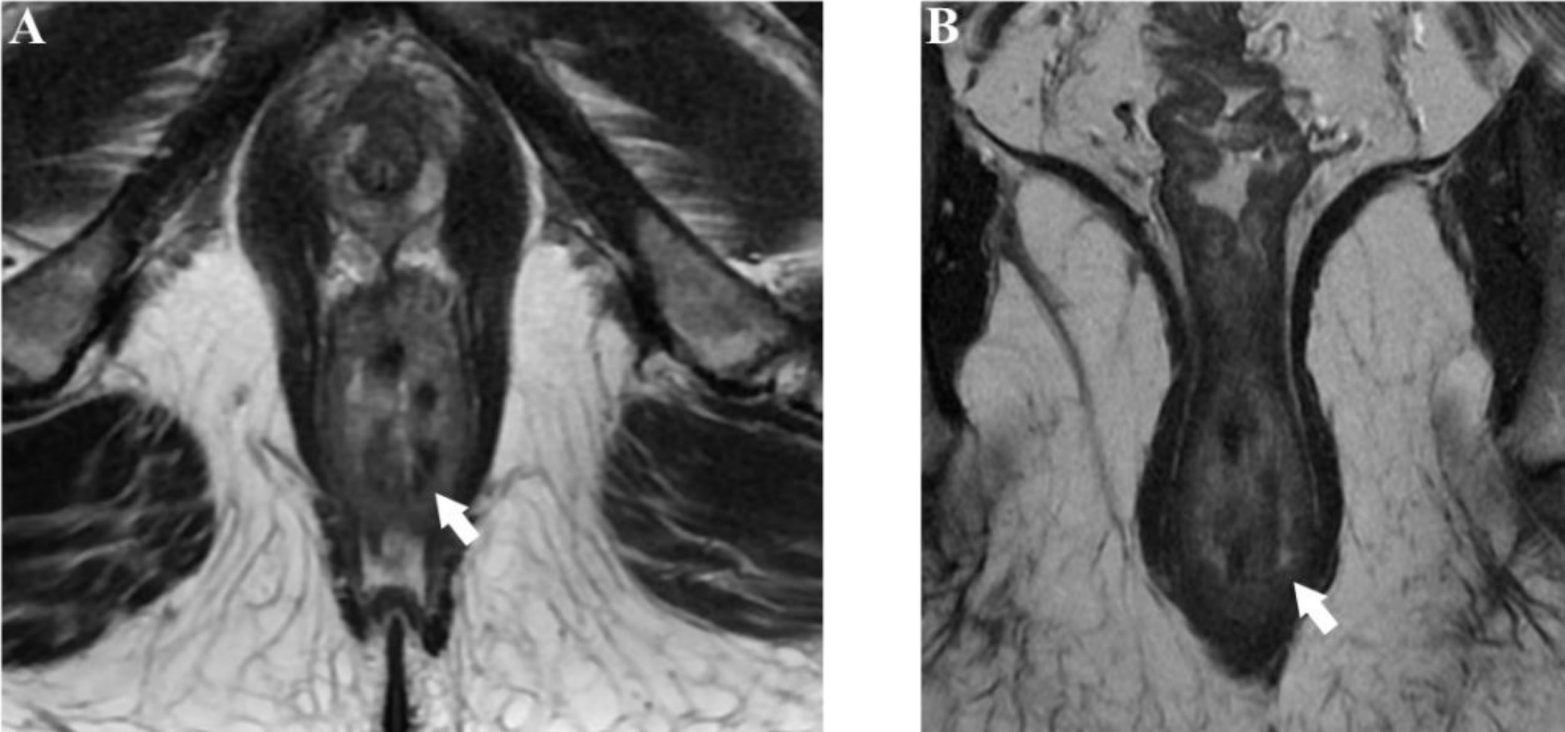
Preoperative perianal magnetic resonance imaging (MRI) of a 43 years-old man. (A) Axial T2-weighted and (B) coronal T2-weighted image images without fat suppression show a mixed hemorrhoid of anal canal (arrow).
